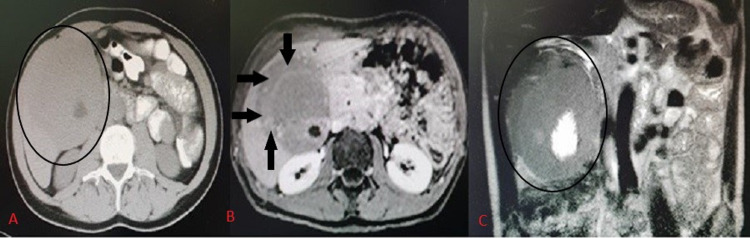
A 15-cm soft GB mass extending to the central liver parenchyma and hepatic hilum without intrahepatic duct dilatation. The big GB mass is included in circles on noncontrast enhanced CT (A) and MRI (C). Extension in liver parenchyma is shown with arrows in MRI of the liver (B). GB, gallbladder
